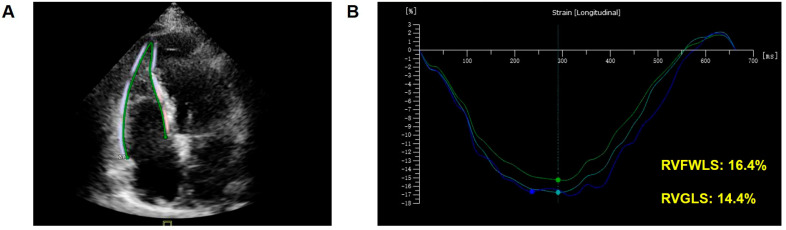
Example of assessment of RV systolic function by 2D-STE in a HT patient. (A) Tracing of 2D-RV longitudinal strain. (B) Processing of 2D-RV longitudinal strain. The green line represents the longitudinal strain of apical-free wall. The dark blue line represents the longitudinal strain of basal-free wall. The light blue line represents the longitudinal strain of mid-free wall. RV: right ventricular; 2D: two-dimensional; STE: speckle-tracking echocardiography; HT: heart transplantation.
